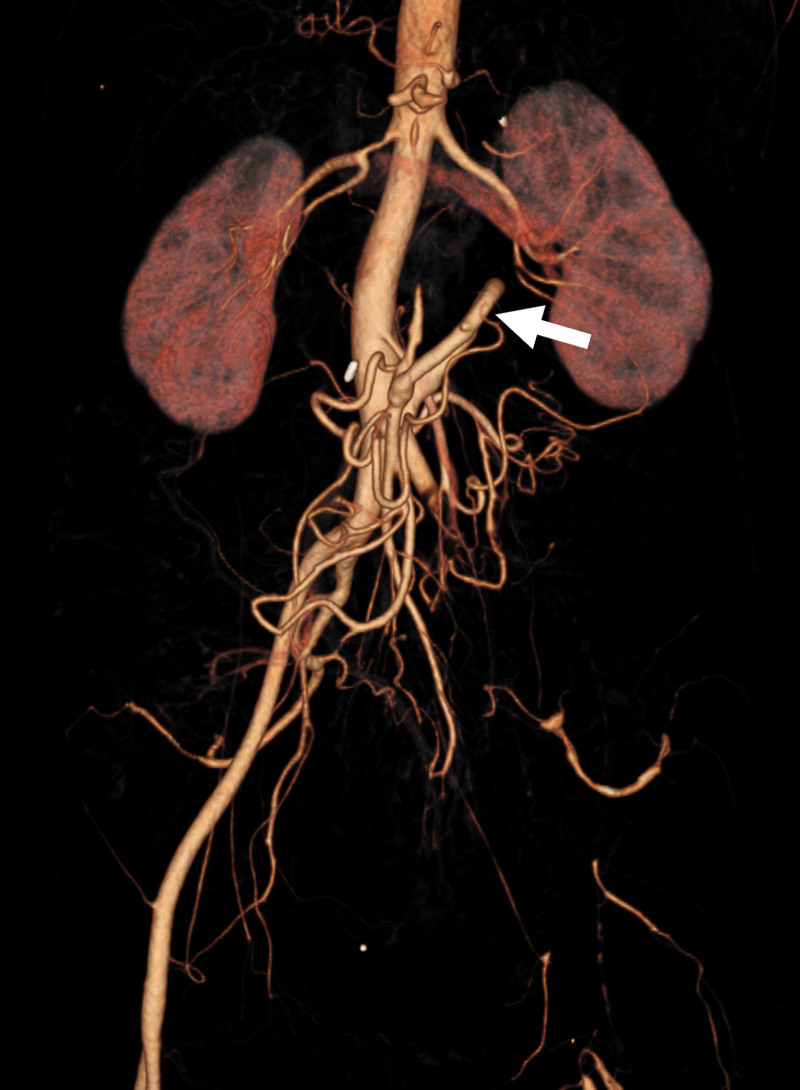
Postoperative computed tomography showing enhanced mesenteric blood flow through the bypass graft (arrow).
